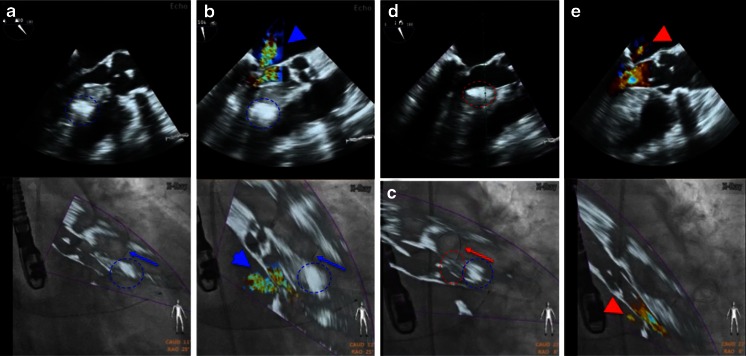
Fusion imaging during transcoronary ablation of septal hypertrophy (TASH). Fusion imaging during alcohol ablation of the second (a, b) and first (c–e) septal branch. a Wire (*blue arrow*) in the second branch after septal alcohol ablation, with the corresponding demarcated area (*blue dashed circle*). This however led to insufficient reduction of left ventricular outflow tract obstruction, as demonstrated by color Doppler imaging (mitral regurgitation due to systolic anterior motion, *blue arrow head* in b). c Placement of the wire into the first septal branch (*red arrow*), corresponding with a septal area (*red dashed circle*) just proximal to that of the second septal branch (*blue dashed circle*). d Demarcation of the proximal septum (*red dashed circle*) after alcohol infusion into the first branch. e Sufficient reduction of left ventricular outflow tract obstruction, demonstrated by markedly reduced mitral regurgitation an flow acceleration
